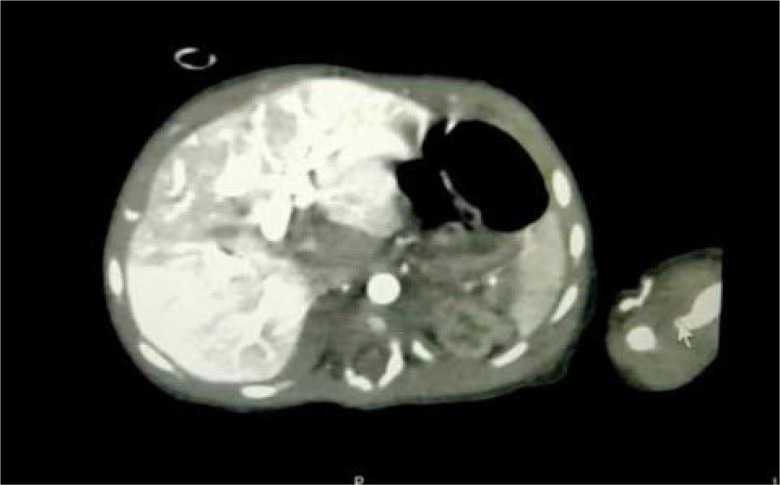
Contrast-enhanced CT scan showing early peripheral enhancement with central progression and central necrotic areas.
